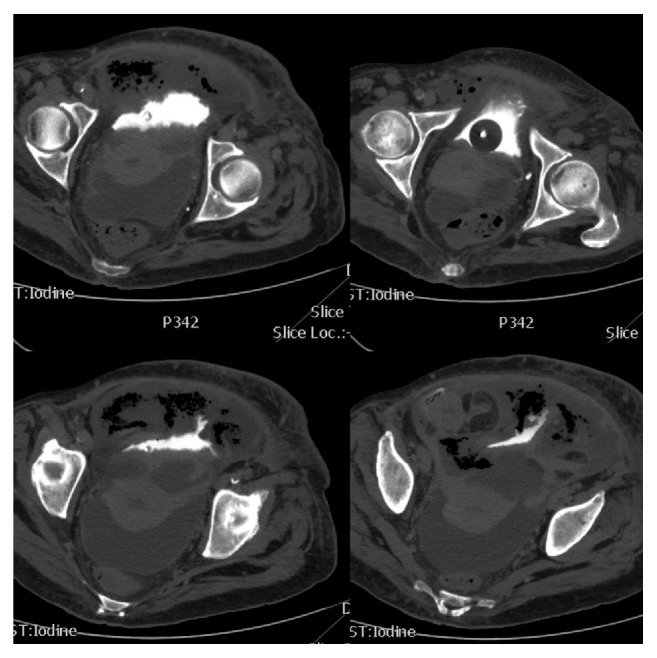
CT cystography revealed extravasation of contrast solution in the peritoneal cavity.
